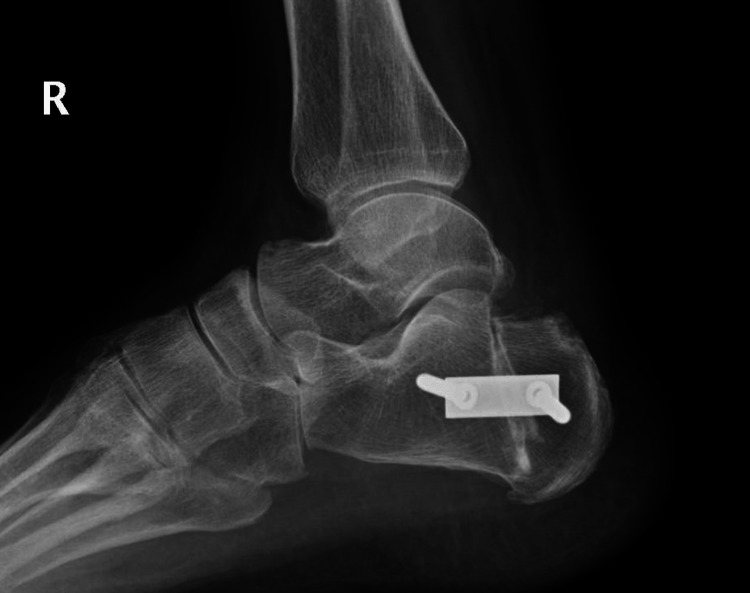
Postoperative radiograph of the same patient after Zadek osteotomy. The posterior prominence has been reduced, and the prominence has been tilted anteriorly.
